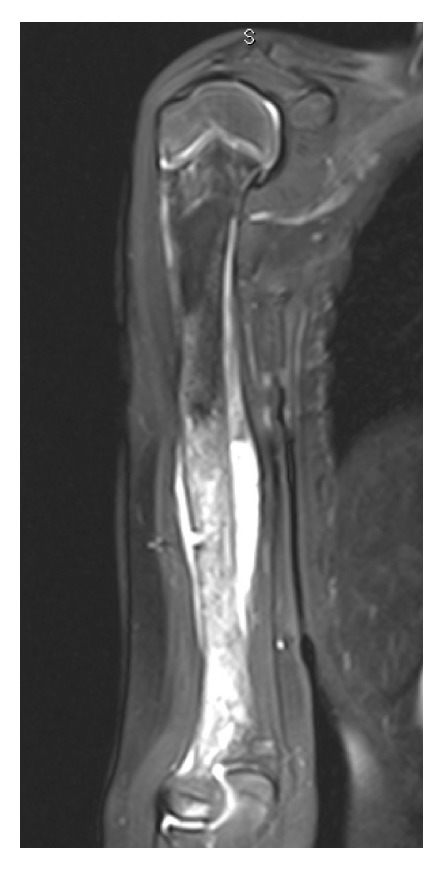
MRI of humerus demonstrating osteosarcoma lesion.
